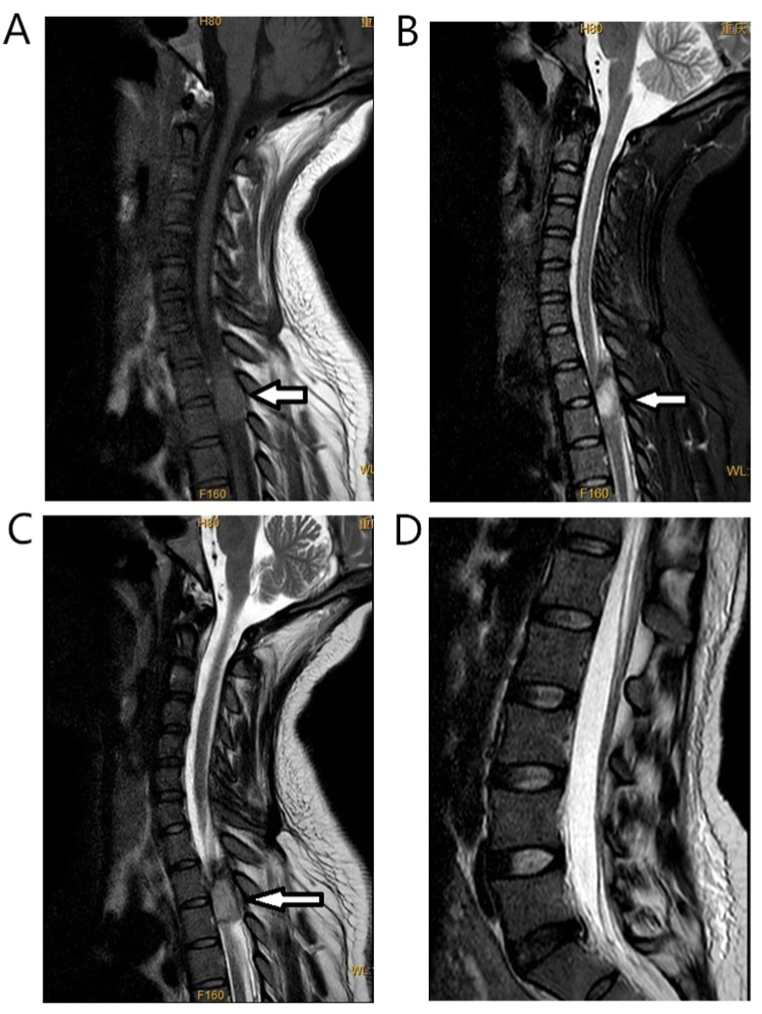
MRI of cervical, thoracic and lumbar vertebrae. Sagittal T2-3 sequence shows epidural abnormality, T1WI and T2FS show high signals (arrows in Fig. 2A and B), and T2WI shows mixed high/low signals (arrow in Fig. 2C). These findings are in agreement with the manifestations of acute epidural hemorrhage. No abnormality was found at the site of lumbar puncture (Fig. 2D).
